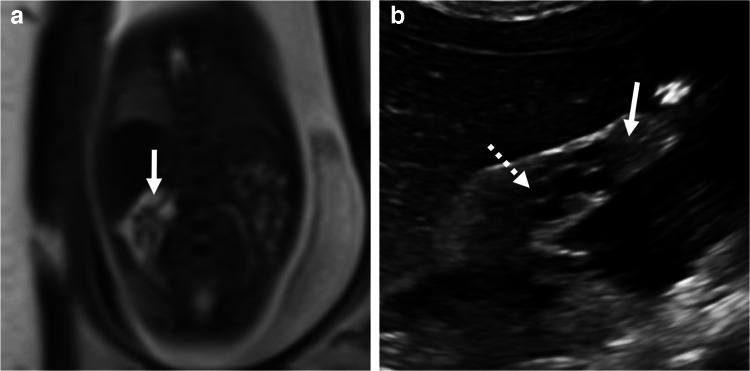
a Coronal Single-Shot Fast Spin Echo (SSFSE) MRI of a 24-week gestation male fetus with postnatal confirmation of a TCF2/HNF1B gene mutation (renal cysts and diabetes syndrome) showing right cystic renal dysplasia and absent left kidney. The right kidney is small with increased T2 signal of the renal parenchyma and numerous small parenchymal cysts (arrow). b Longitudinal renal ultrasound at day one of life showing a small echogenic right kidney with poor corticomedullary differentiation (arrow) and multiple medullary cysts (dotted arrows)
